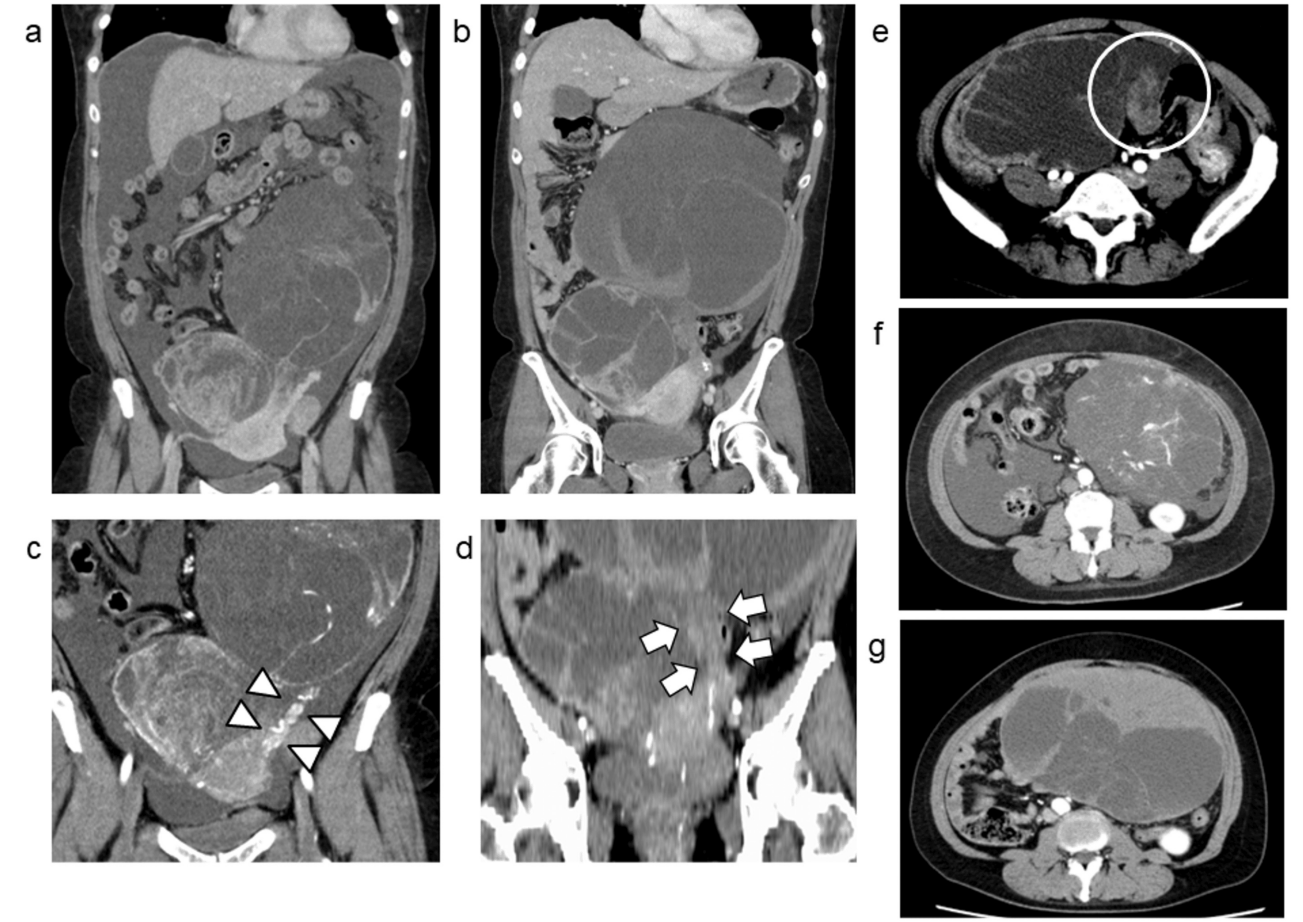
Changes on CT images before and after torsion Coronal (a-d) and axial (e-g) contrast-enhanced CT images in the venous phase (a, b) and arterial phase (c-g). Swollen left and right ovarian tumors are present after torsion (b) compared to before torsion (at initial diagnosis) (a). Contrast enhancement is visible in the artery within the left infundibulopelvic ligament (arrowheads) before torsion (c). After torsion, there is an absence of enhancement in the ovarian and/or infundibulopelvic ligaments (arrows) (d). A twisted pedicle sign is indicated (circle) (e). Contrast enhancement is observed before torsion (f). Following torsion, contrast enhancement is absent, and a relatively high-attenuation area ventral to the tumor suggests hemorrhage or edema (g).
